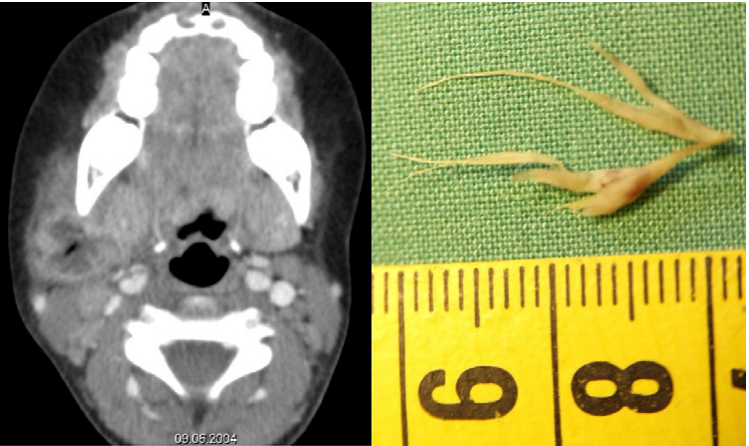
a: Computed tomography (CT) of the cervical abscess. b: Extracted foreign body. A grass blade of 2 cm of length.
